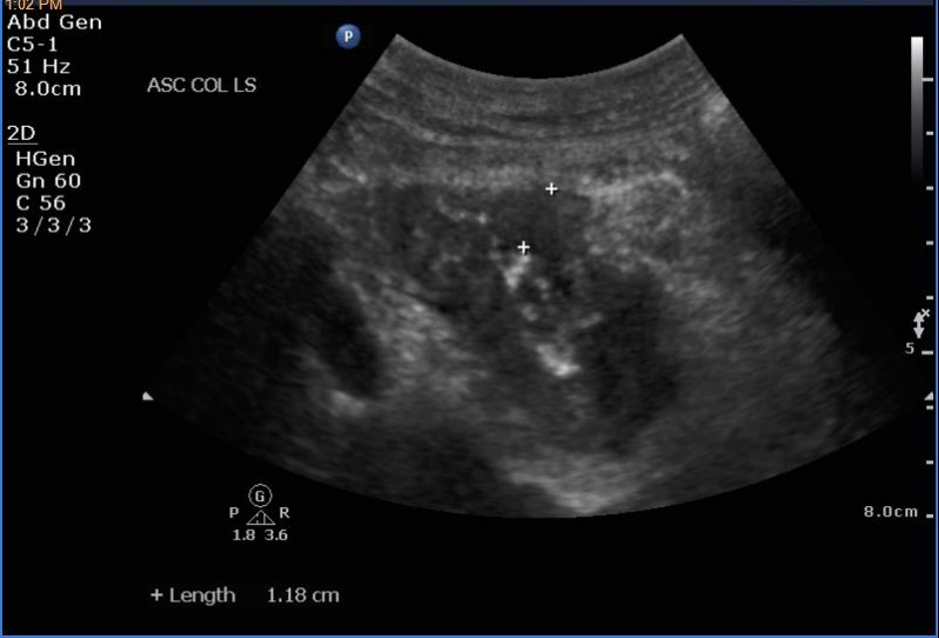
Ascending colon carcinoma. Probe place at right hypochondrium, probe marker pointing to patient’s head. Hypoechoic irregular circumscribed wall thickening (11.8mm) around the hyperechoic pattern of bowel lumen presenting with a kidney-shaped appearance by sonography. There is also loss of echo-stratification and compressibility.
